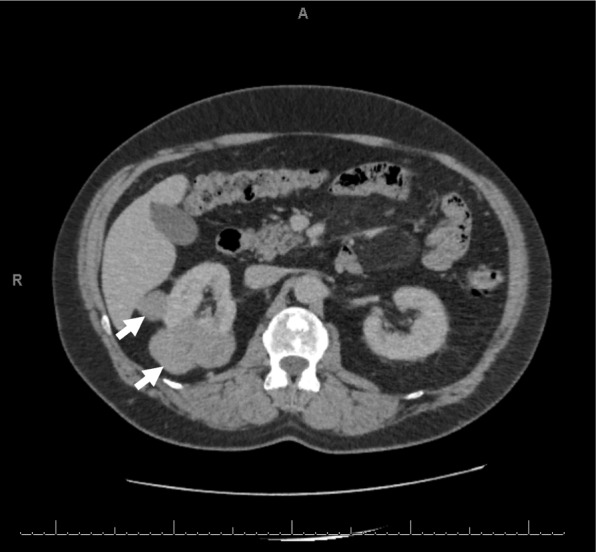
Computed tomographic scan of kidneys showing tumor in the right kidney (*white arrows*) prior to nephrectomy
